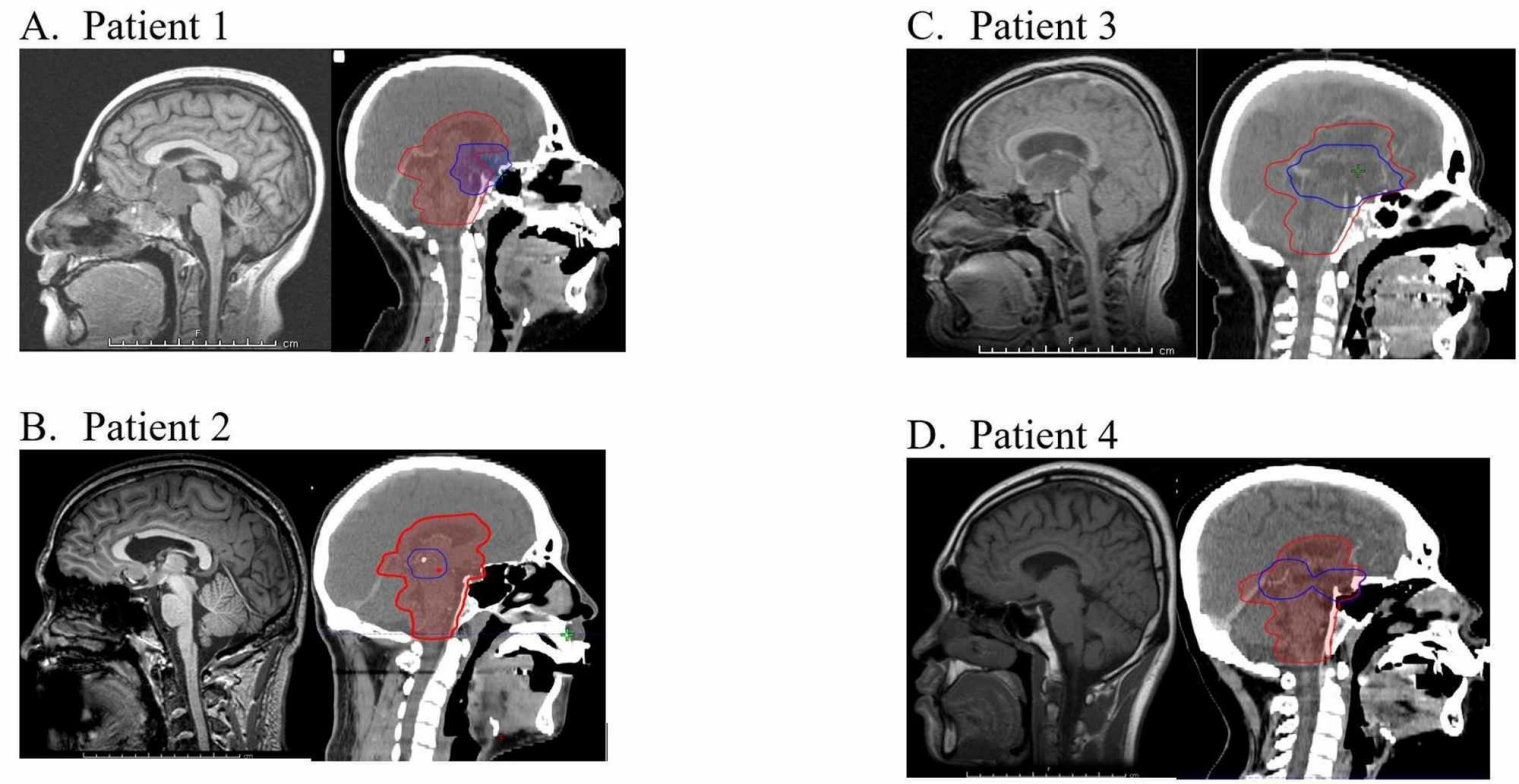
Tumor locations for four patients A-D. Sagittal MRI (left) and Eclipse TPS images with target contours – blue for PTV; red for WVV-PTV (right) TPS: treatment planning system; PTV: planning target volume; WVV: whole ventricular volume Eclipse: Varian Medical Systems, Palo Alto, CA
